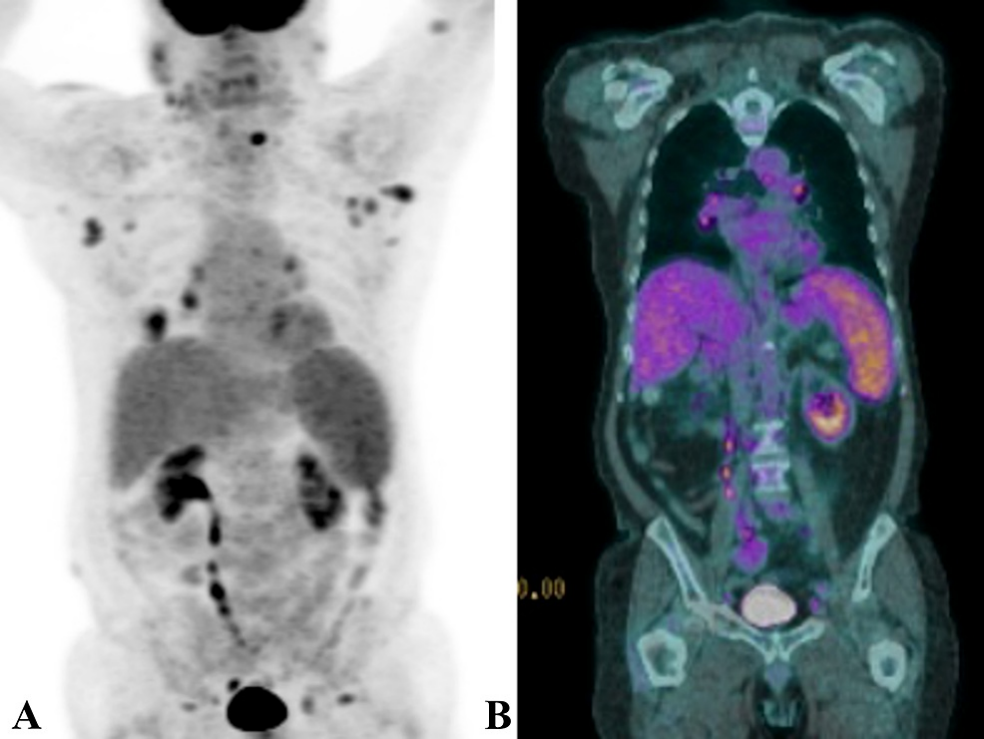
ENKTL relapse following standard of care chemotherapy Radiographic findings at the time of ENKTL relapse following initial treatment with GDP chemotherapy are seen on a (A) positron emission tomography (PET) scan with a three-dimension (3D) reconstruction revealing multiple hot spots reflecting active disease and on a (B) PET-computerized tomography (PET-CT) revealing slice-by-slice sites of active disease. ENKTL, Extra-nodal natural killer T-cell lymphoma.
